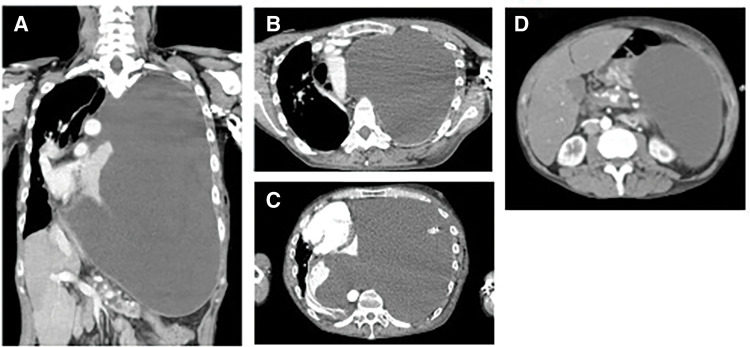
Contrast-enhanced CT scans at hospital admission. (A–C) CT images showing massive left pleural effusion and mediastinal shift to the right. No apparent communication between the pancreatic pseudocyst and the thoracic cavity is detected on CT. (D) Abdominal CT showing pancreatic calcifications consistent with chronic pancreatitis and a suspected pancreatic pseudocyst.
